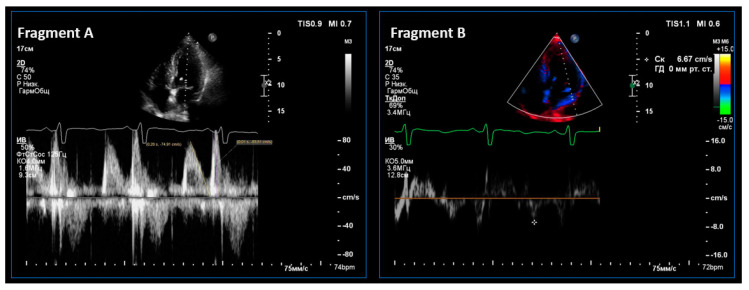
An episode of echocardiography in Participant A. (male, 55 years old). In the Doppler mode (Fragment A), transmitral blood flow is shown with an assessment of Peaks E and A. In the tissue, the Doppler mode and the fibrous ring of the mitral valve movement are shown in the left ventricle early-filling phase.
